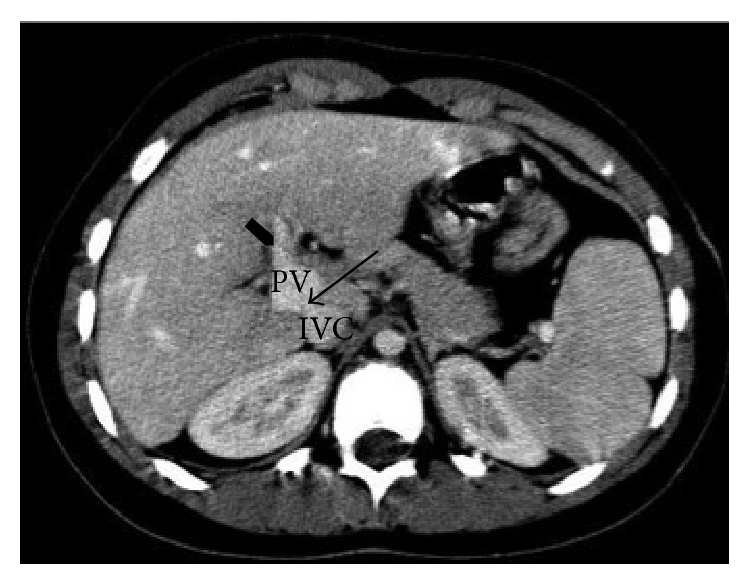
Axial contrast-enhanced CT images. The arrow shows the shunt between the portal vein (PV) and the inferior cava vein (ICV); at the hepatic hilum, the PV appears enlarged with only one intrahepatic portal branch (arrowhead).
